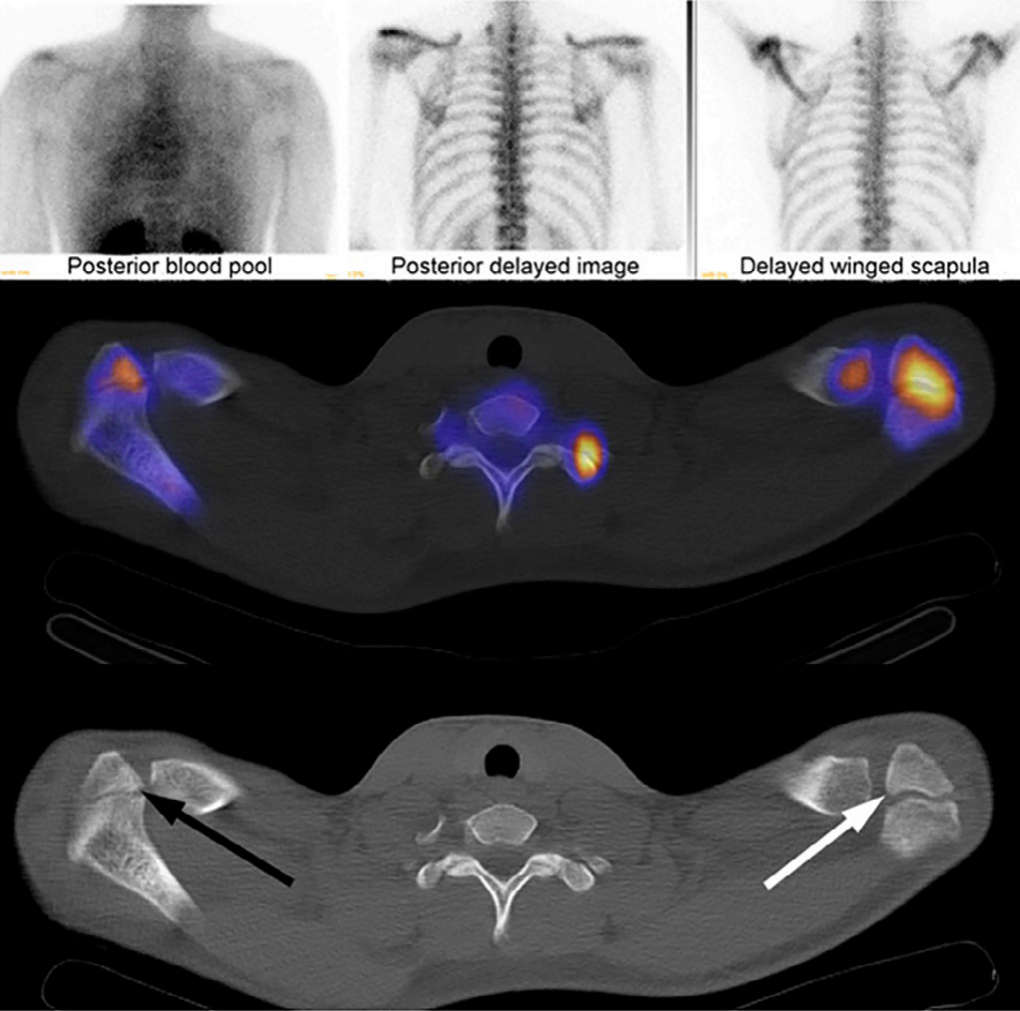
Top row shows static bone scan and blood‐pool images, middle row images shows fused SPECT/CT images, and bottom row shows attenuation correction CT. Arrows are placed at ossification centers of apophyses. Center is at meso‐acromion on the left (white arrow) but at preacromion on the right (black arrow). The right apophysis is nearly fused and has less activity than the unfused apophysis of the left acromion^34^.
